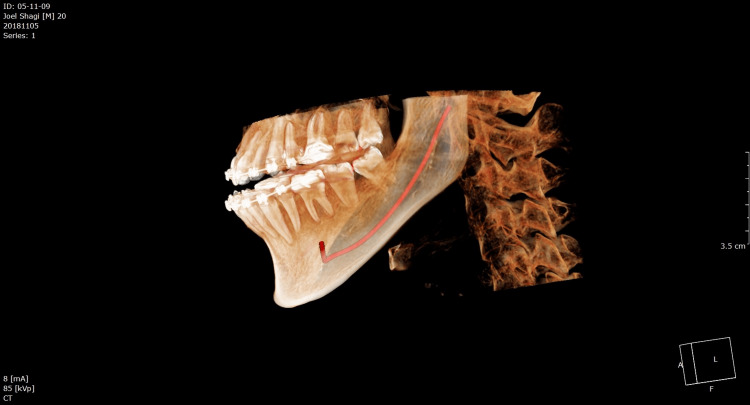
CBCT imaging of the post-operative BSSO setback CBCT: cone-beam computed tomography, BSSO: bilateral sagittal split osteotomy
